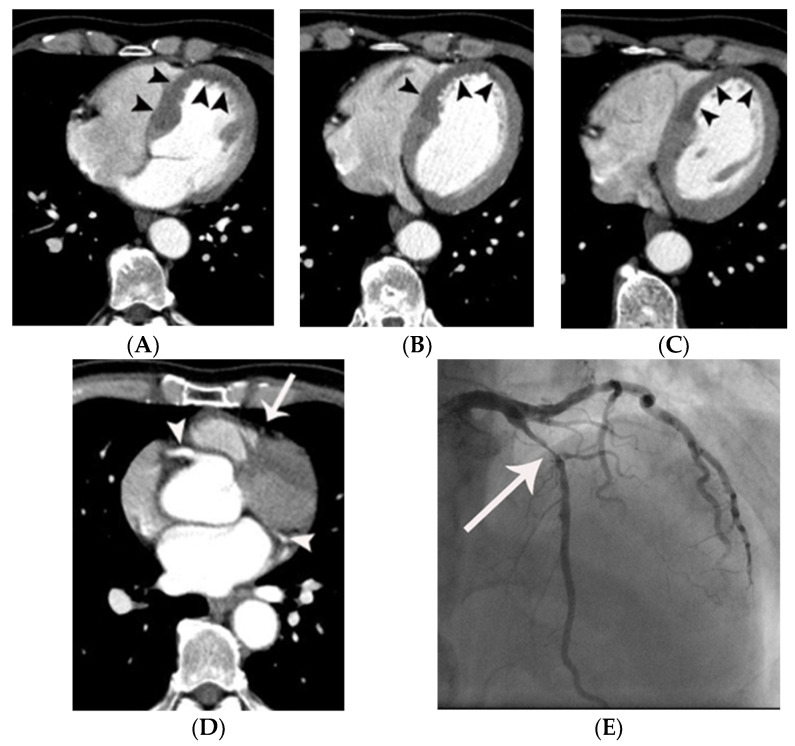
Representative case, demonstrating probable MPD in a 69-year-old male with acute chest pain. Subendocardial or transmural hypoattenuation (arrowheads in (A–C)) was demonstrated on the multiple non-gated axial CT images along the left anterior descending coronary territory (i.e., antero-septum and the left ventricular apex). Note that attenuation of the mid-left anterior descending coronary artery (arrow in (D)) is low compared to the right coronary artery and left circumflex coronary artery (arrowheads in (D)), suggesting critical narrowing or occlusion of the left anterior descending coronary artery. Critical narrowing in the proximal left anterior descending coronary artery was confirmed on subsequent coronary angiography (arrow in (E)).
